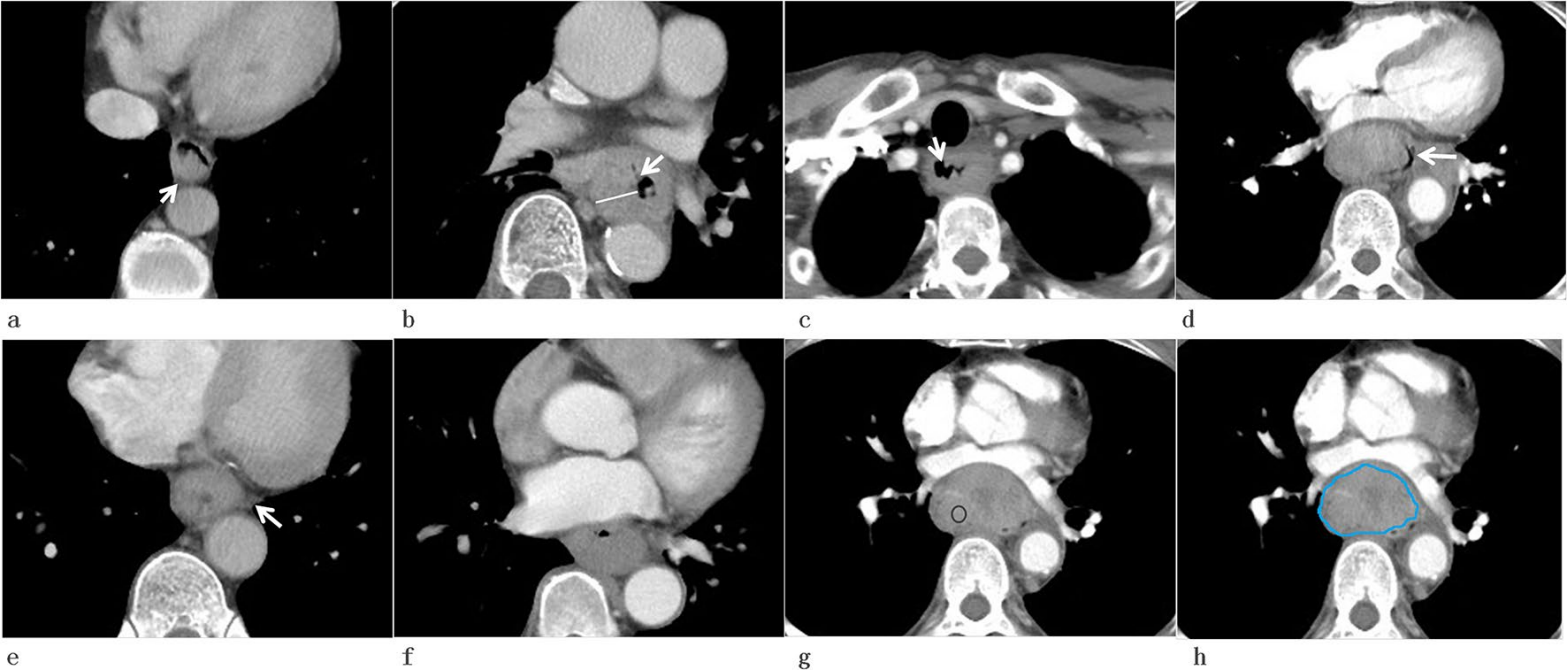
CT signs of esophageal tumor. a One focal esophageal lesion with tumor range of 0–1/2 (arrow). b One diffuse type lesion with tumor range of 1/2–1 with deep ulcer in anterior wall (arrow), the white line showed the measurement of thickness of tumor. c One diffuse growth lesion with non-smooth tumor air surface (arrow). d One expansive esophageal lesion with smooth tumor air surface (arrow). e One esophageal lesion with fibrosis of peritumoral fat space (arrow). f One esophageal lesion with homogeneous intensity. g, h One esophageal lesion with heterogeneous intensity with ROI of maximum enhanced intensity (g) and ROI of encompassing the tumor on the maximal section (h)
